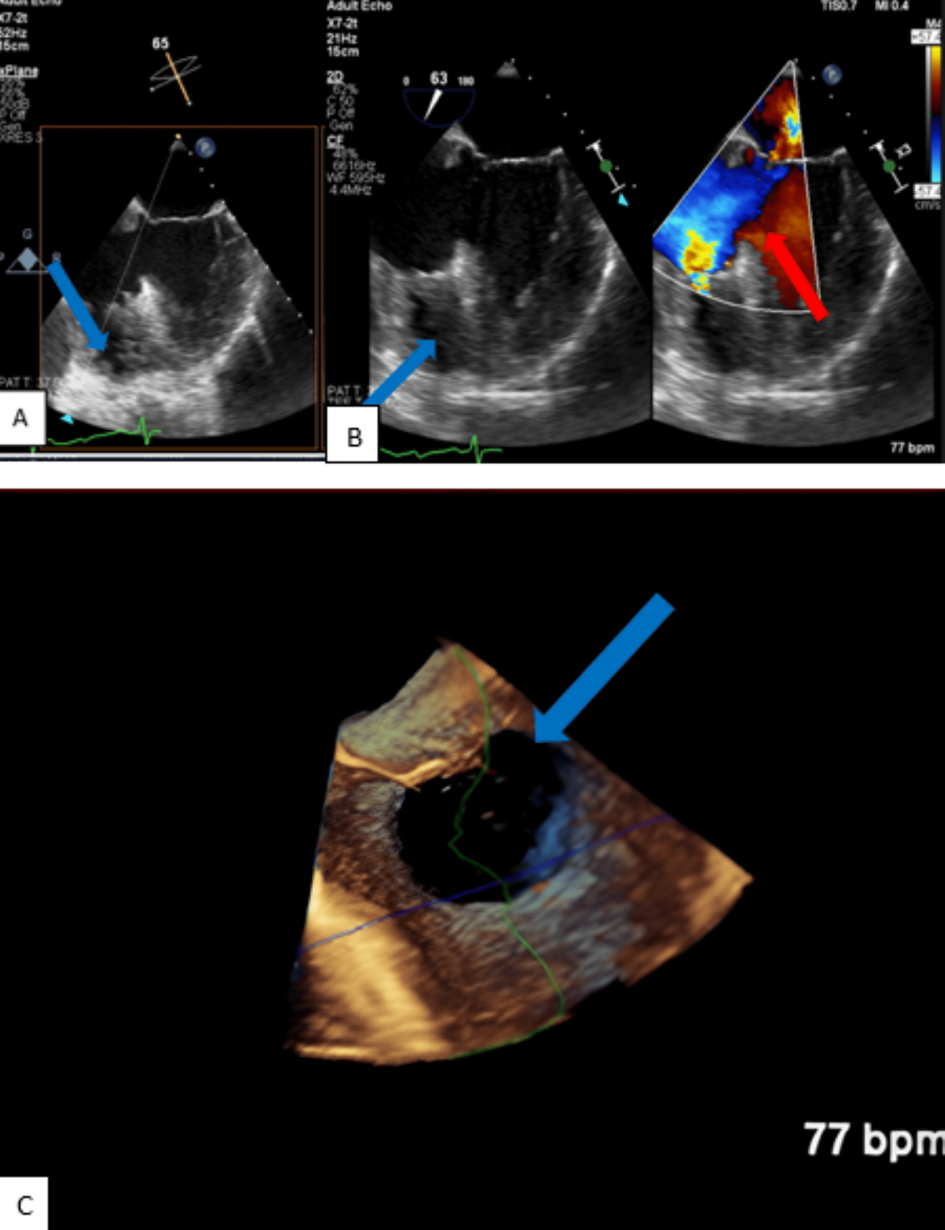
(A) TEE demonstrating an aneurysmal sac protruding into the right ventricle (blue arrow); (B) TEE demonstrating the presence of contained rupture into the right ventricle distal to the tricuspid annulus (blue arrow) with color doppler ultrasound revealing turbulent flow (red arrow); (C) Three-dimensional TEE revealing the 3.55 x 3.79 cm orifice (blue arrow). TEE: Transesophageal echocardiogram
